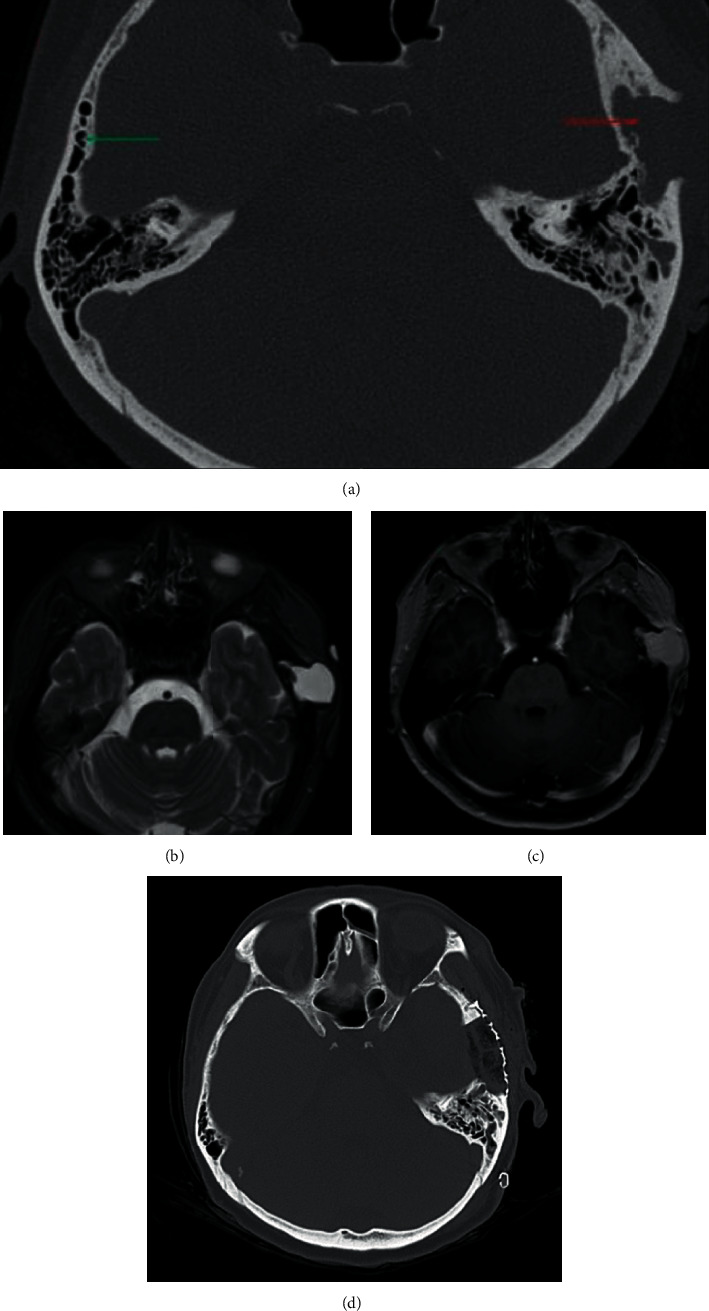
(a) Axial CT of the temporal bones showing a cystic mass of the left squamosal temporal bone disrupting the lateral cortex on the left (red arrow) and a well-pneumatized contralateral squamosal bone (green arrow). (b) T2-weighted axial MRI showing a fluid-filled mass at the left squamosal temporal bone. (c) T1 imaging reveals the lesion to be hyperintense and nonenhancing. (d) Postoperative CT showing complete resection with reconstruction via mesh cranioplasty.
